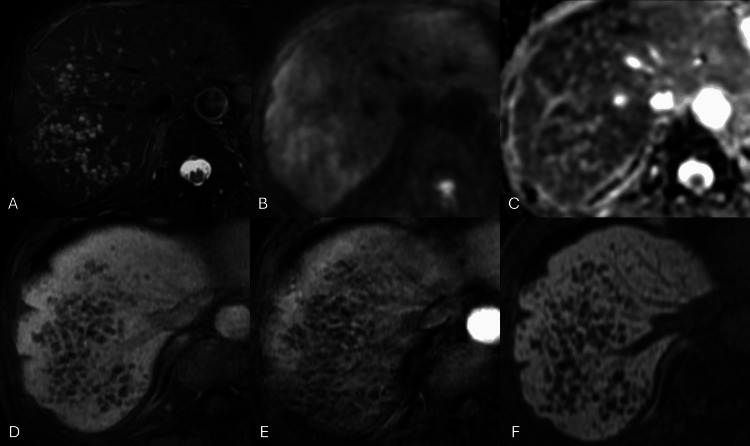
MRI of peliosis hepatis There are multiple cystic lesions with high intensity on fat-suppressed T2-weighted imaging with fluid-fluid levels (A) and absence of restricted diffusion (B: diffusion-weighted imaging, C: apparent diffusion coefficient map). Fat-suppressed T1-weighted imaging shows hypointensity (D) without contrast enhancement in the arterial phase (E) or EOB uptake in the hepatobiliary phase (F) on dynamic MRI using Gd-EOB-DTPA. MRI, magnetic resonance imaging; Gd-EOB-DTPA, gadolinium ethoxybenzyl diethylenetriaminepentaacetic acid
